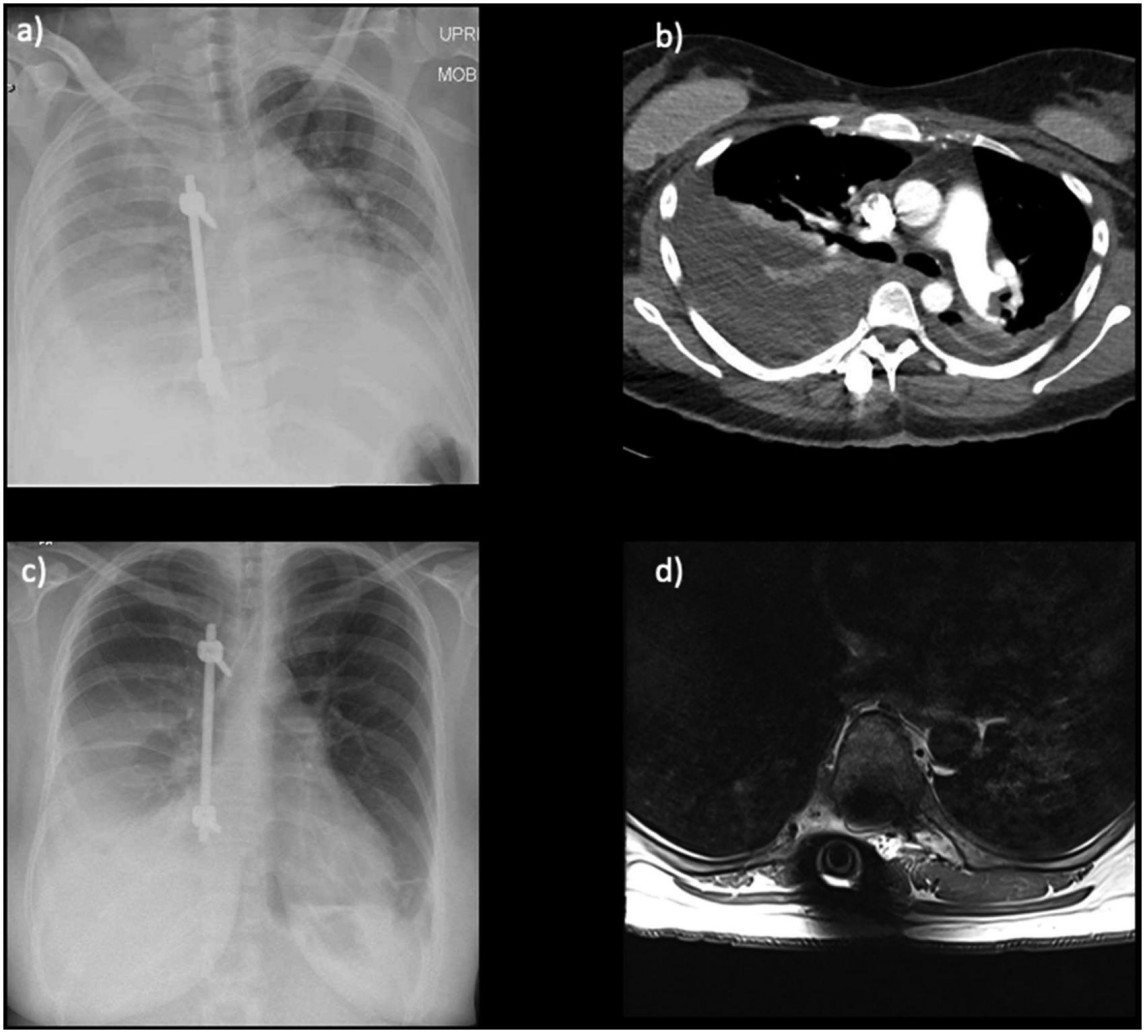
Case of benign thoracic spine tumour with immediate post-operative chest x-rays (a) and CTPA (b) showing pleural effusion. After a period of Liquoguard-driven lumbar drainage, a repeat chest x-ray 5 days later (c) shows interval improvement, and a delayed MR 4 months later shows resolution of effusion at the lung bases (d).
